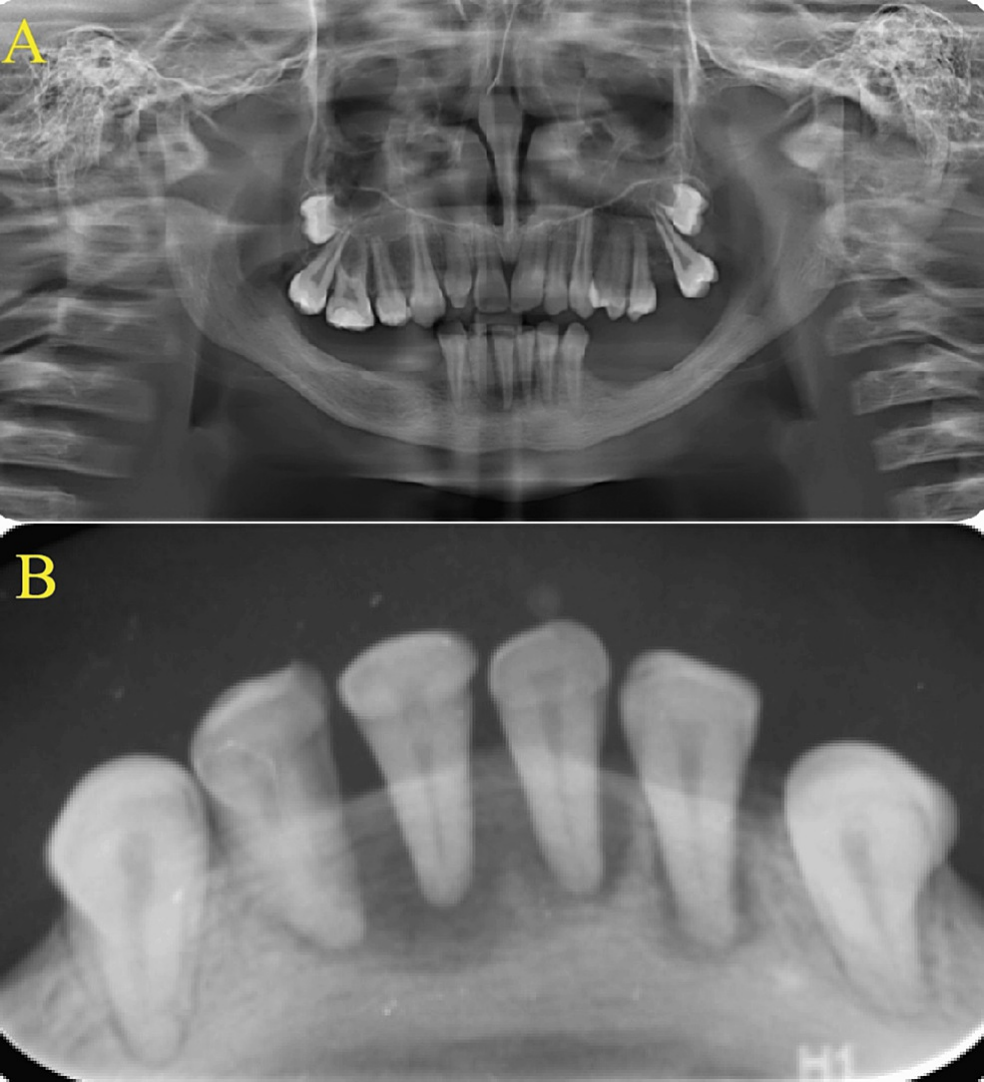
Radiographic assessments (A) panoramic radiograph; (B) periapical radiographs of lower anterior teeth
